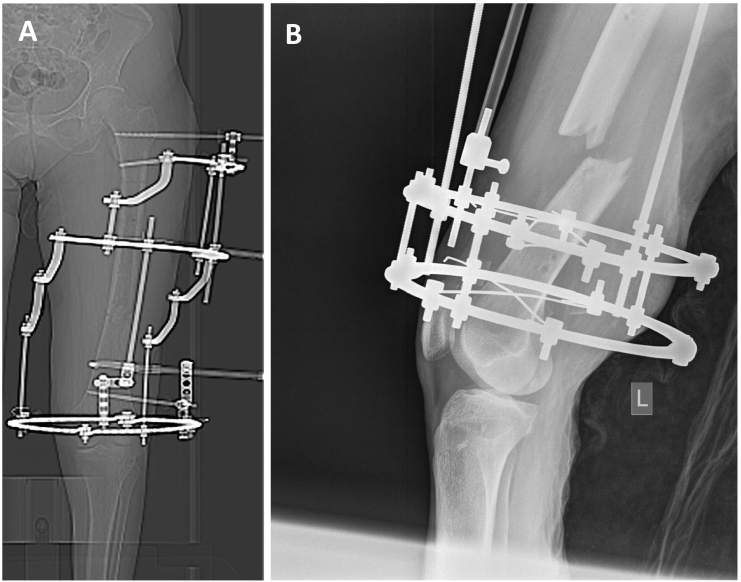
CT-overview scan (A) and lateral X-ray (B) of the distal left femur with external hybrod fixation and without any signs of callus formation four months after the initial trauma.
